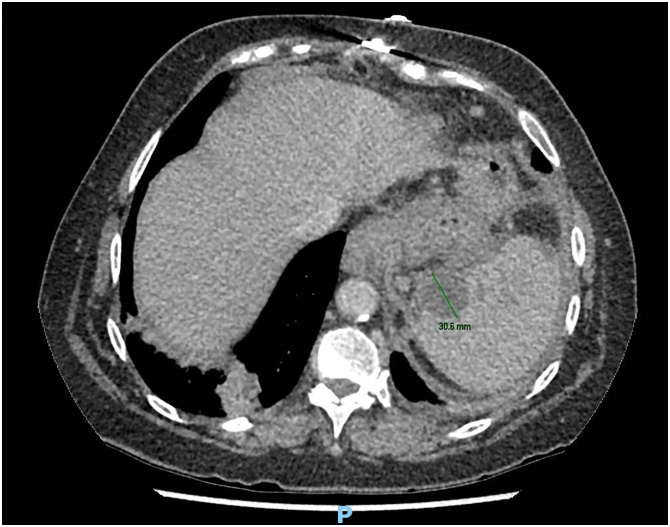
Abdominal computed tomography showing an axial view of the splenic abscess.
